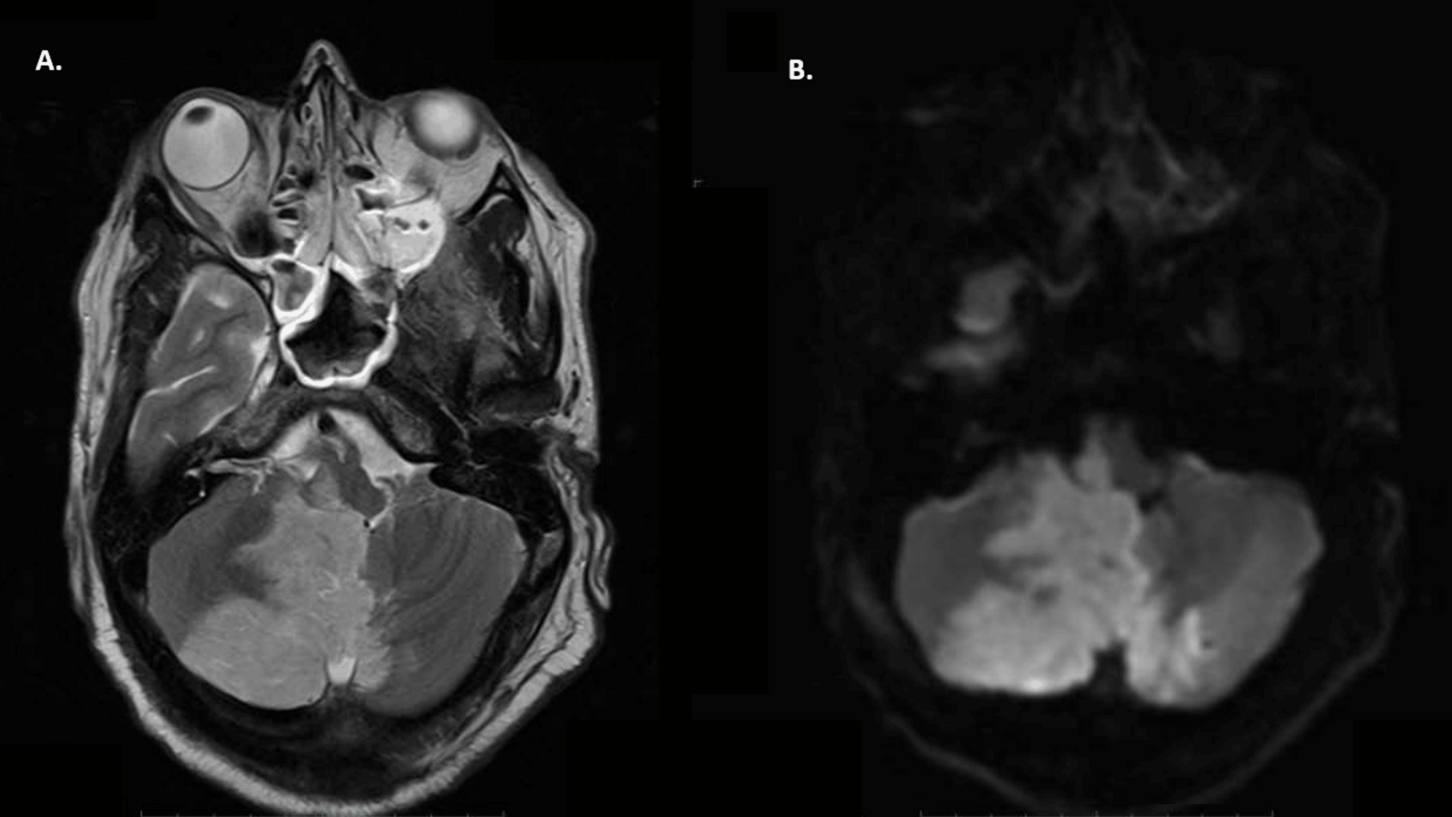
MRI Brain (A): T2 axial section at the level of medulla showing hyperintense signal in right lateral medulla along with right cerebellar hemisphere; (B) DWI axial section showing areas of diffusion restriction in the same territory suggestive of acute infarct
